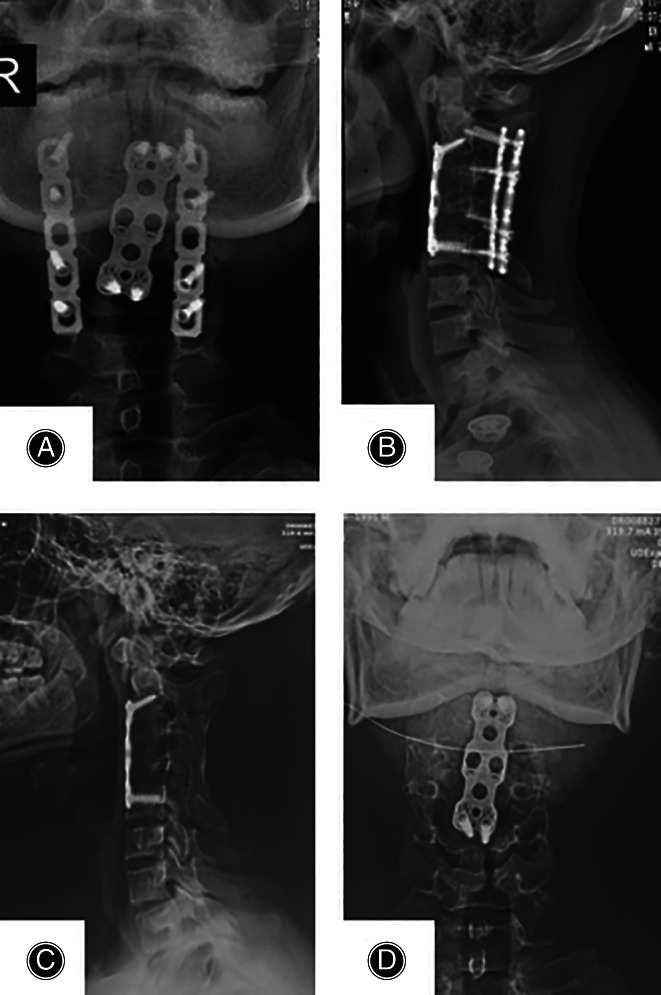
Conventional radiology: Postoperative AP and lateral view at 2.5‐year before posterior implant removal (A and B) showing cervical alignment and bone union; AP and lateral radiographs at the last follow‐up indicating an optimal cervical spine correction and bone union (C and D).
